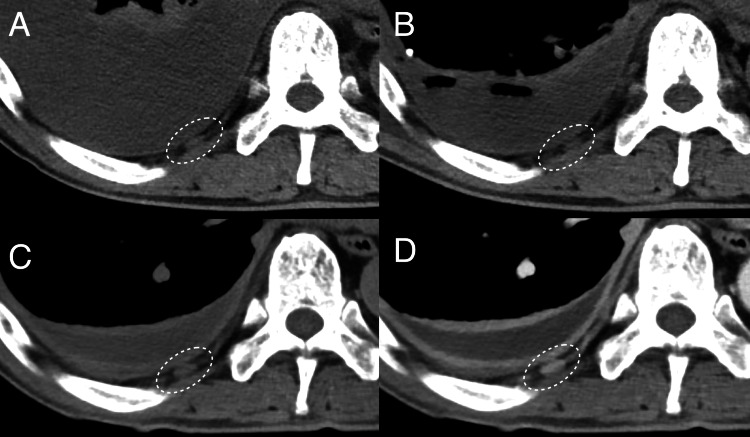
Close-up views of CT scans corresponding to Figures 1A-1D. (A, B) Close-up views before anti-tuberculosis therapy showing mild intercostal lymphadenopathy at the right ninth rib (dotted circles). (C, D) Close-up views 27 days after the initiation of anti-tuberculosis therapy, showing enlargement of the right ninth intercostal lymph node (dotted circles).
